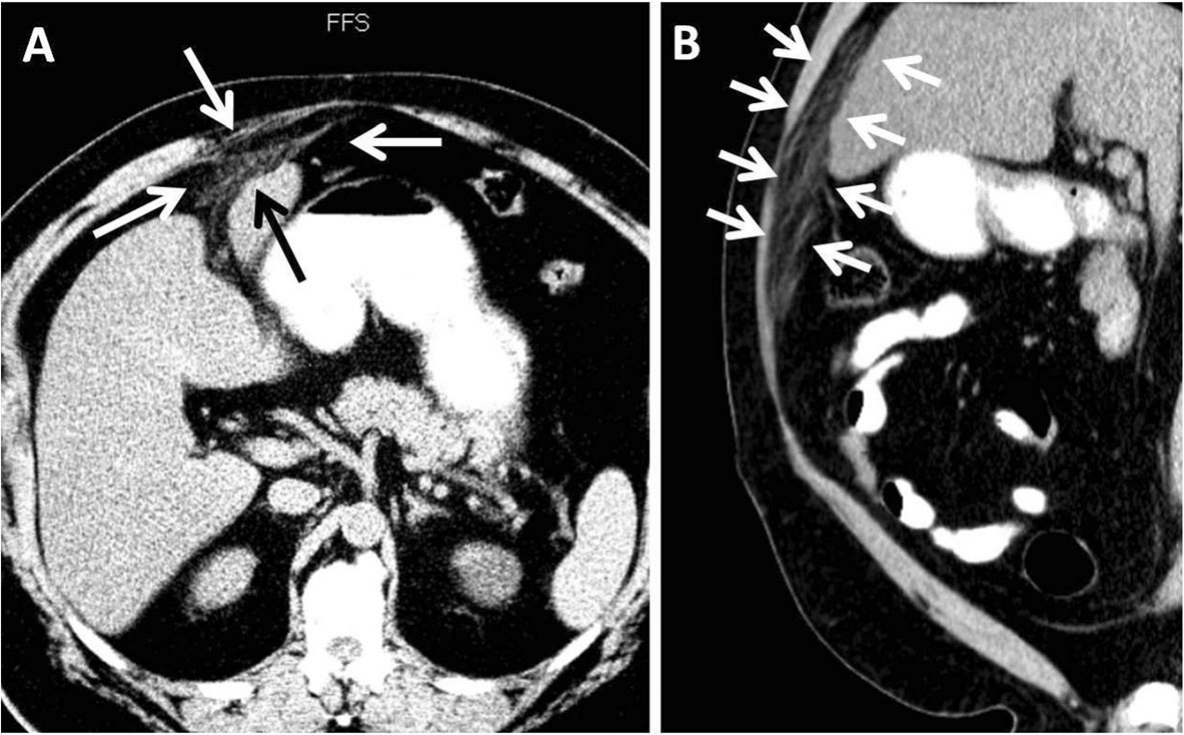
Axial (a) and sagittal (b) computed tomographic images showing fat stranding extending (arrows) from the umbilical fissure toward the umbilicus
